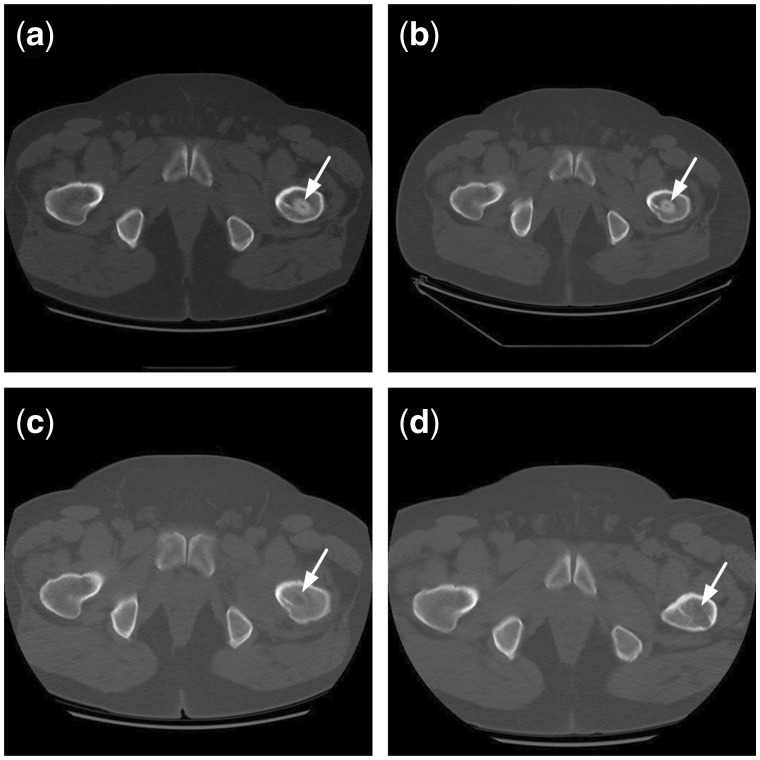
CT changes after implantation of the reconstructional rod. (a) CT in 3 months on postoperative; (b) CT in 5 months on postoperative; (c) CT in 9 months on postoperative; (d) CT in 11 months on postoperative
